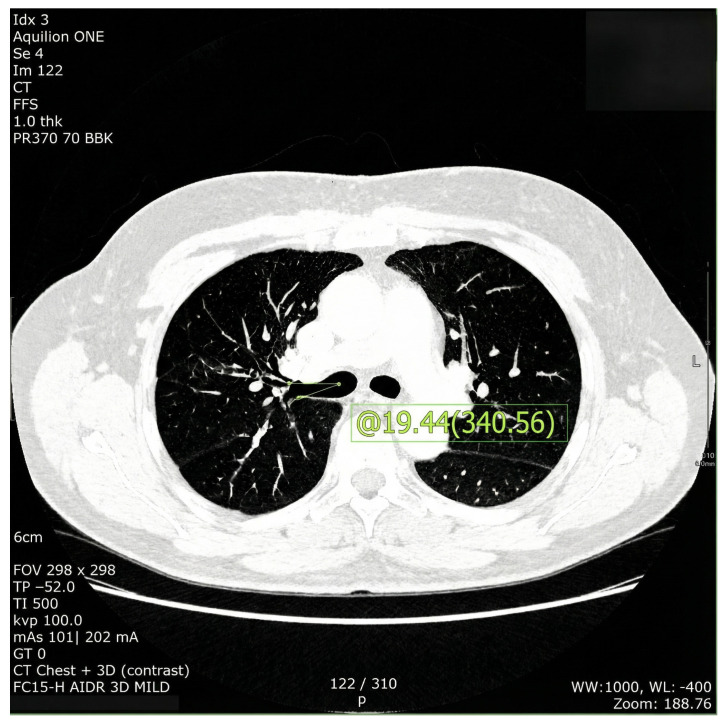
Axial image of chest computed tomography selected for measurement of angle between the horizontal midline of the right main bronchus and the center of the right upper lobe orifice.
